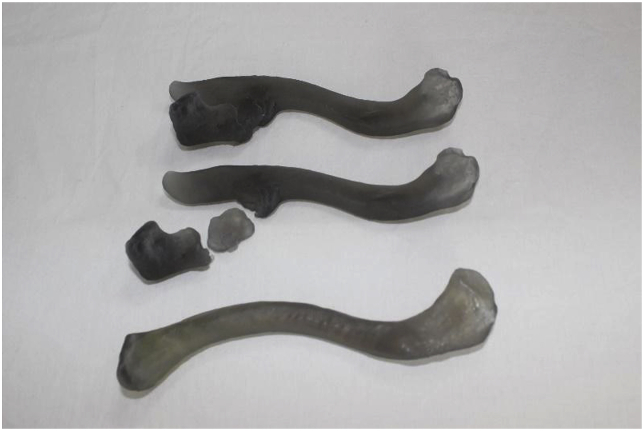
Three-dimensional models created from computed tomography scan data. The two models at the top are the affected-side clavicles. The model at the bottom is the mirror image of the contralateral clavicle.
